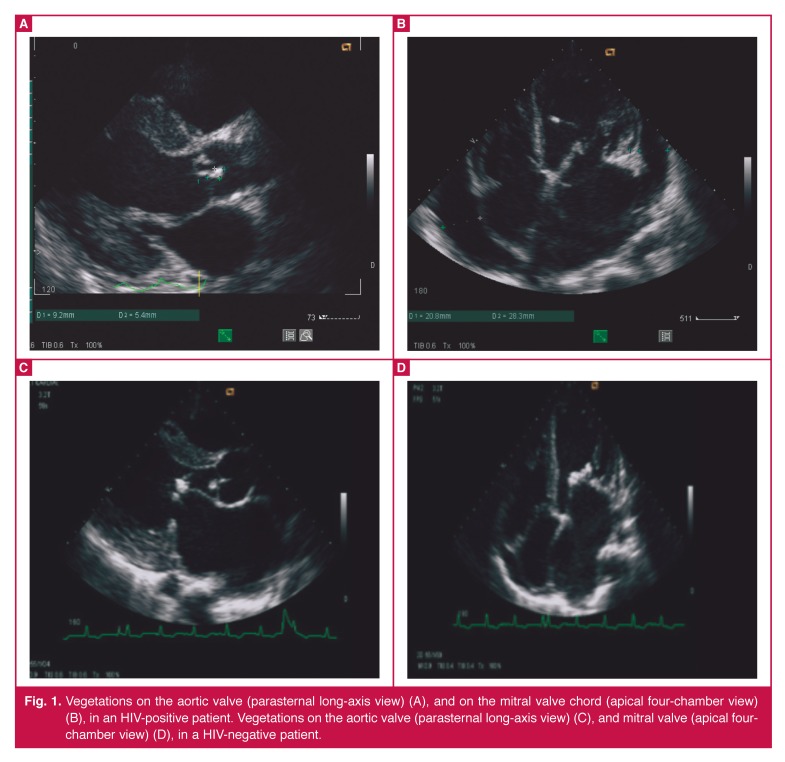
Vegetations on the aortic valve (parasternal long-axis view) (A), and on the mitral valve chord (apical four-chamber view) (B), in an HIV-positive patient. Vegetations on the aortic valve (parasternal long-axis view) (C), and mitral valve (apical fourchamber view) (D), in a HIV-negative patient.
